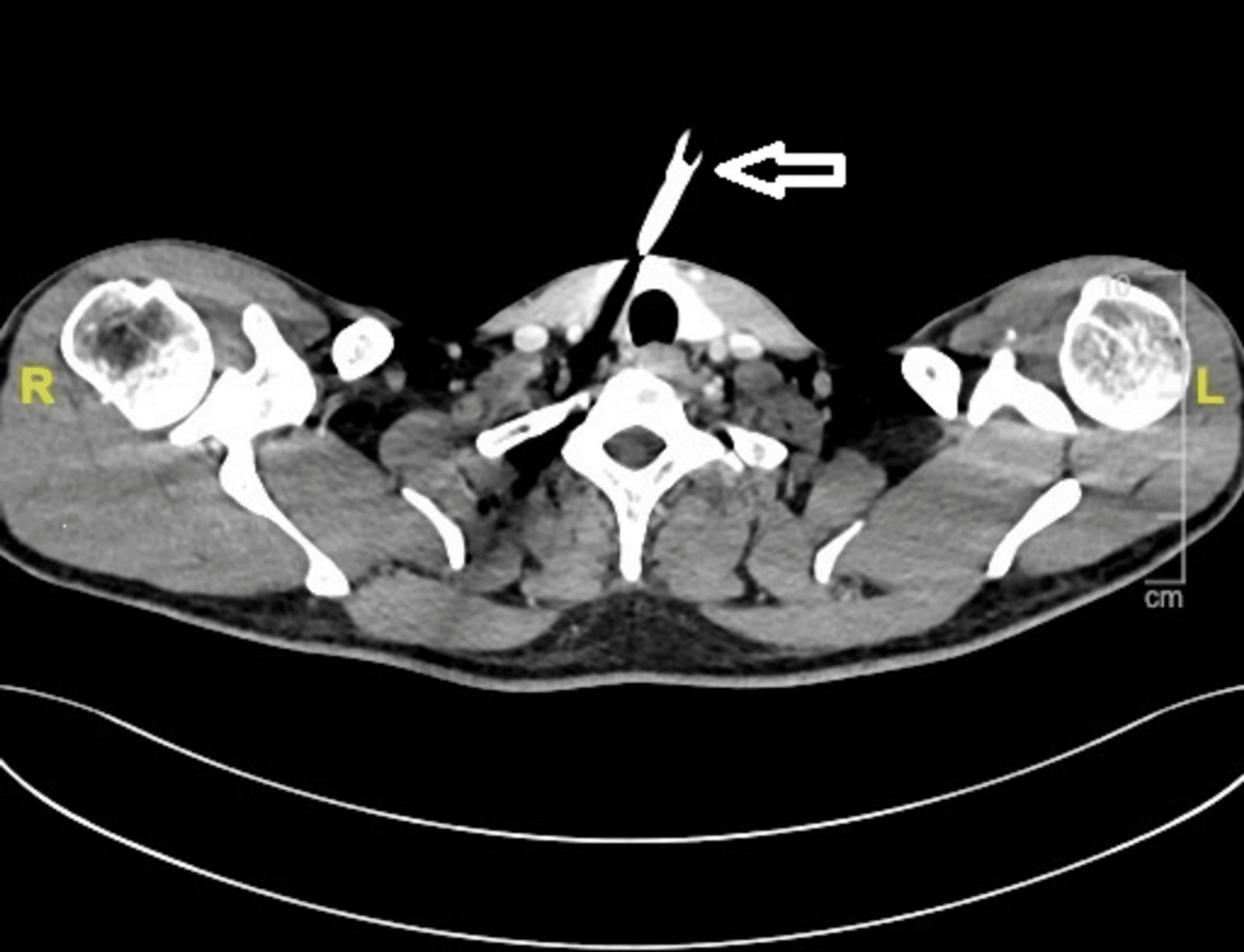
CT image depicting the knife trajectory (white arrow) through the sternohyoid muscle and thyroid gland, extending toward the apical pleura, and sparing major vascular structures. CT, computed tomography
